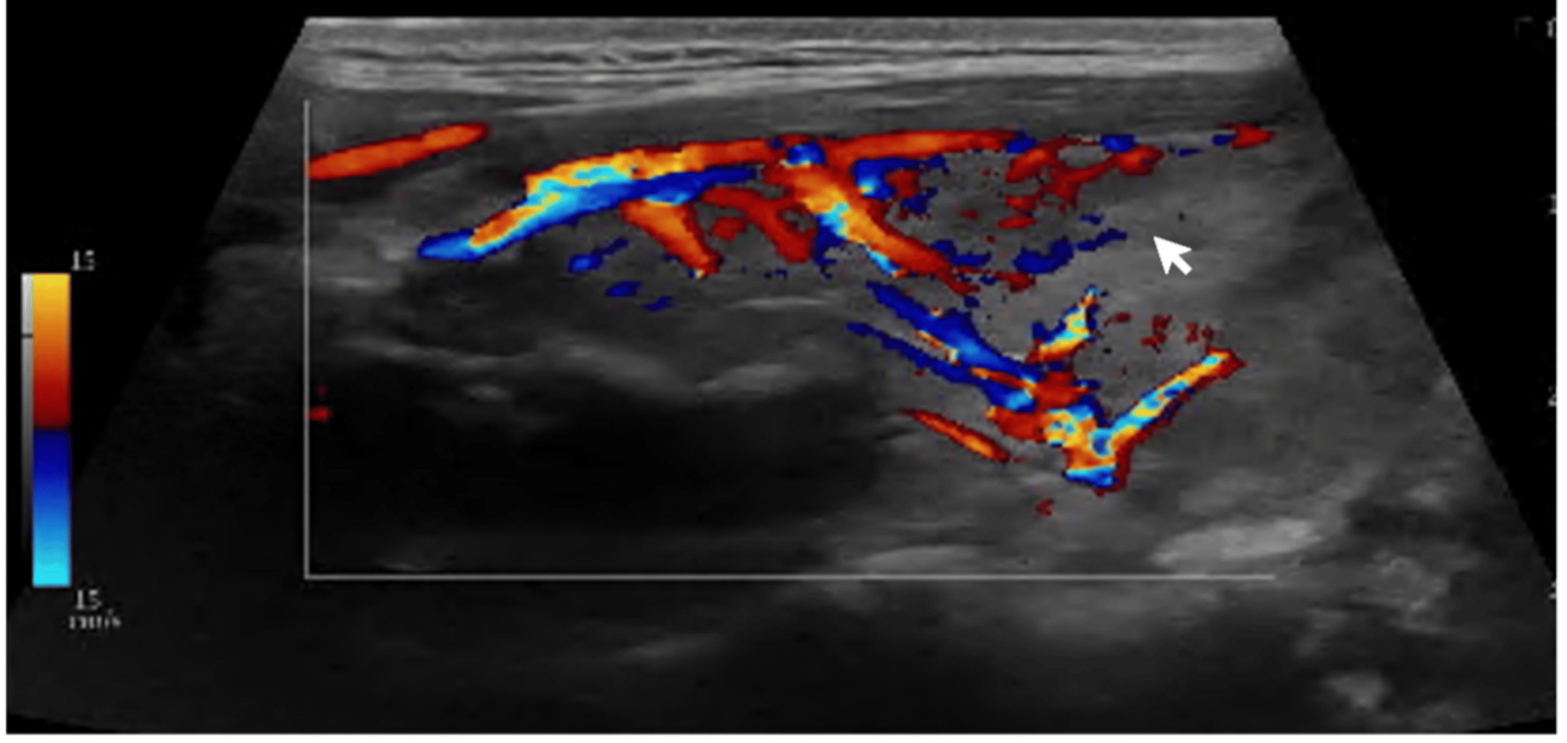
Doppler ultrasound-thyroid (imaging) The Doppler ultrasound image demonstrates an increased vascularity (white arrow).
